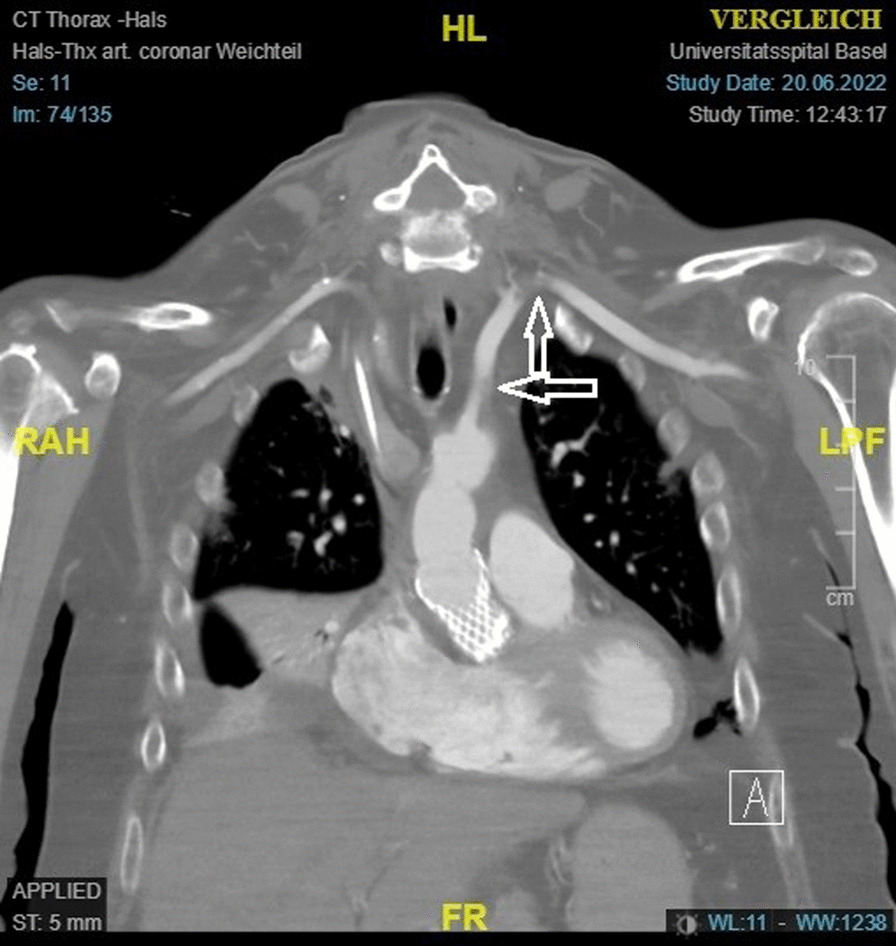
Postoperative CTA scan showing a dissection flap in the proximal left subclavian artery and contrast loss just distal to the junction of the left vertebral artery
